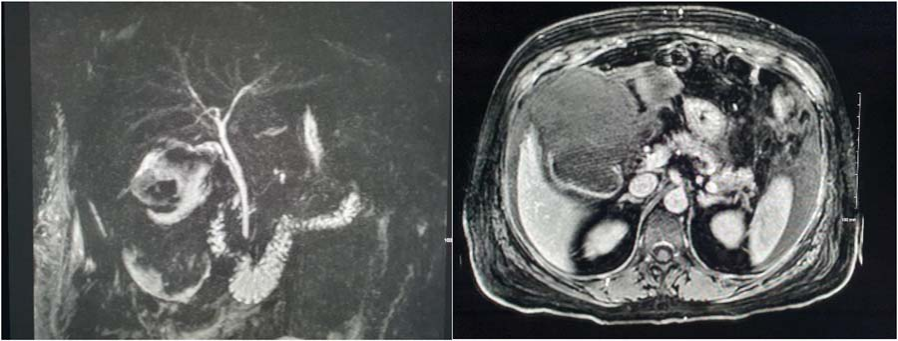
MRCP and post contrast MRI images demonstrating the wide mouthed frank disruption of gallbladder wall.
